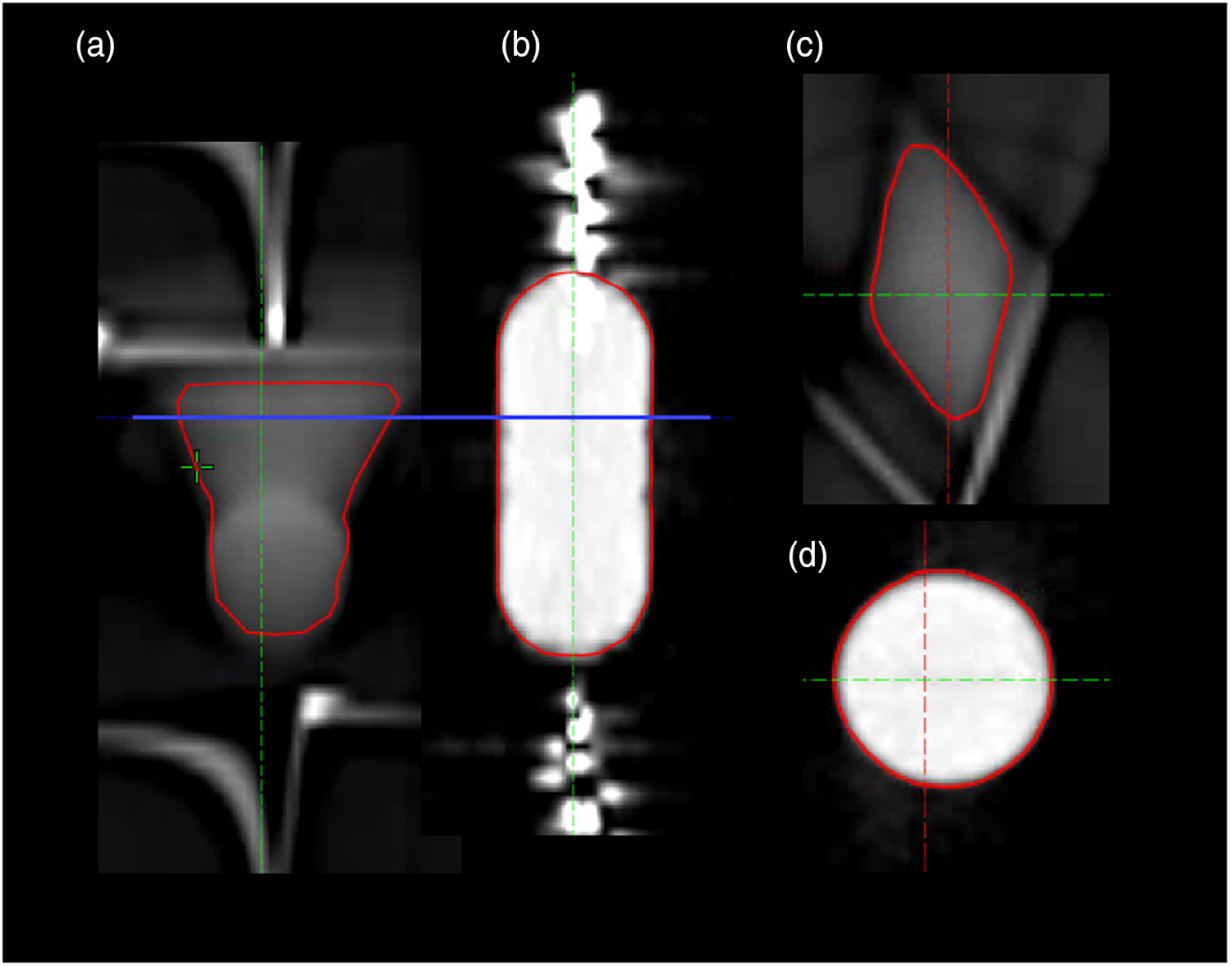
Comparison of target visualization for sinusoidal motion with 15 mm amplitude. (a) Sagittal view from HyperSight CBCT with contoured ITV. (b) Sagittal view from 4DCT MIP reconstruction. (c) Axial slice from HyperSight, and (d) axial slice from 4DCT MIP, both corresponding to the location indicated by the blue line in (a) and (b).
